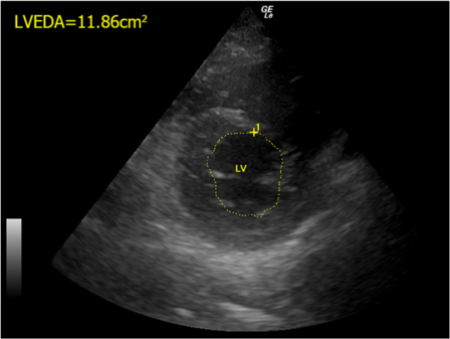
The normal LVEDA measurement. The LVEDA is measured at the level of mid-papillary level of left parasternal short axis view in a normal human being. (*LVEDA* left ventricular end diastolic area, *LV* left ventricle)
